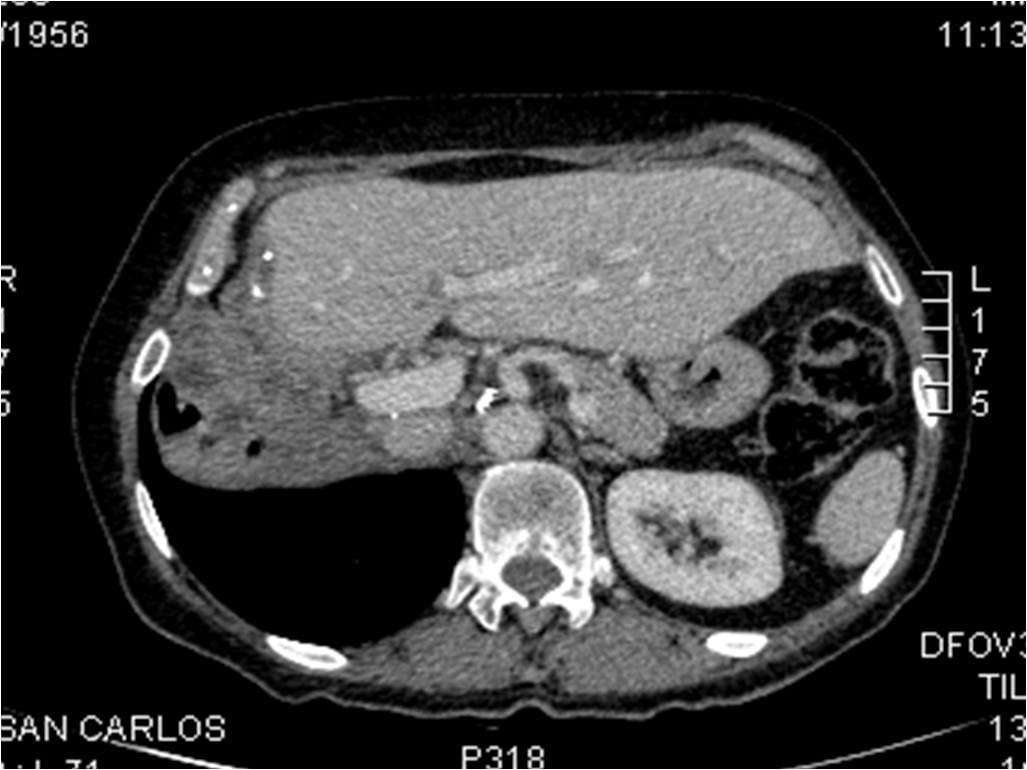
Abdominal CT scan after extensive surgery
